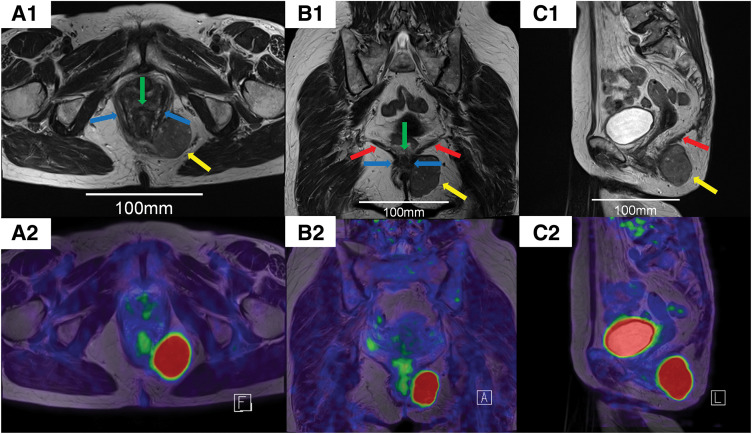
MRI and fused PET-MRI of the tumor. T2-weighted MRI scans are shown in the (A1) axial, (B1) coronal, and (C1) sagittal planes. Corresponding fused PET-MRI images are shown in the (A2) axial, (B2) coronal, and (C2) sagittal planes. A well-defined 46 × 28 mm mass is noted on the left dorsolateral aspect of the lower rectum, which is T1-hypointense, T2-hyperintense, and demonstrates intense fluorodeoxyglucose accumulation, which was in contact with the puborectal muscle. The continuity with the rectum was unclear. The tumor is indicated by the yellow arrow, the rectum by the green arrow, the puborectal muscle by the blue arrows, and the levator ani muscle by the red arrows.
